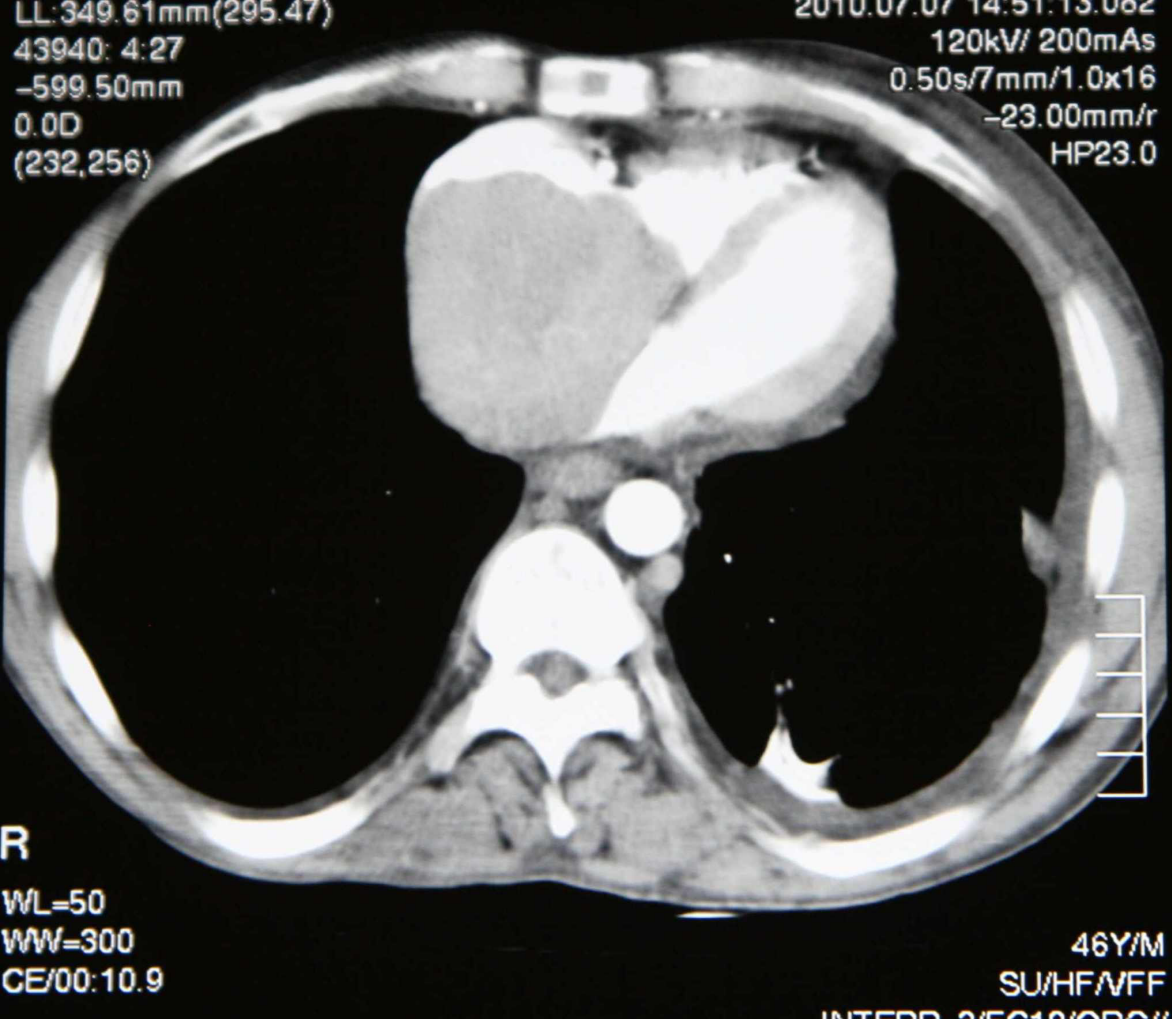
CT angiogram verifying the presence of a mass inside the right atrium occupying almost the whole cavity.
